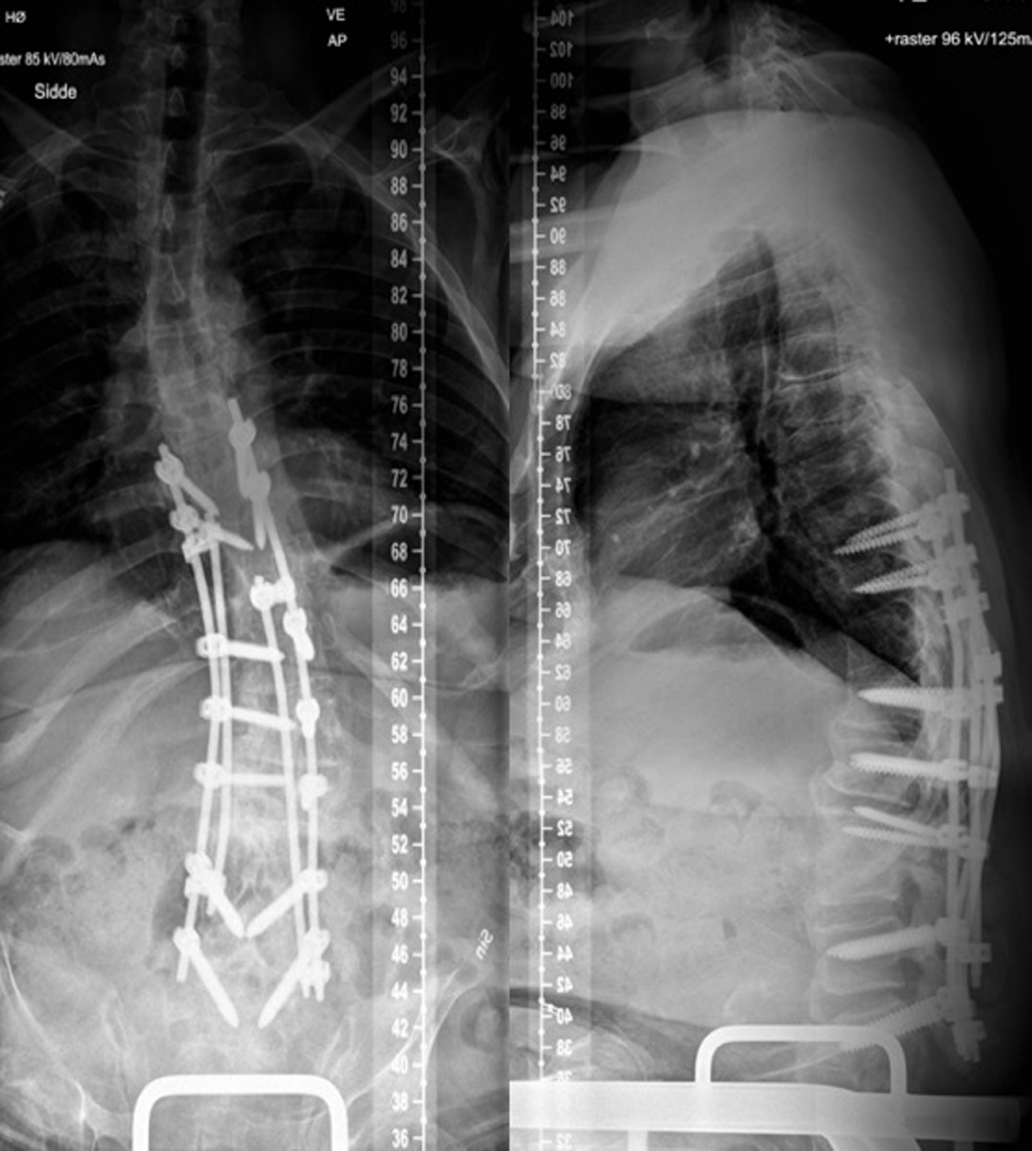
1 year follow up radiograph shows good consolidation at the pseudoarthrosis site and correction of the deformity.
